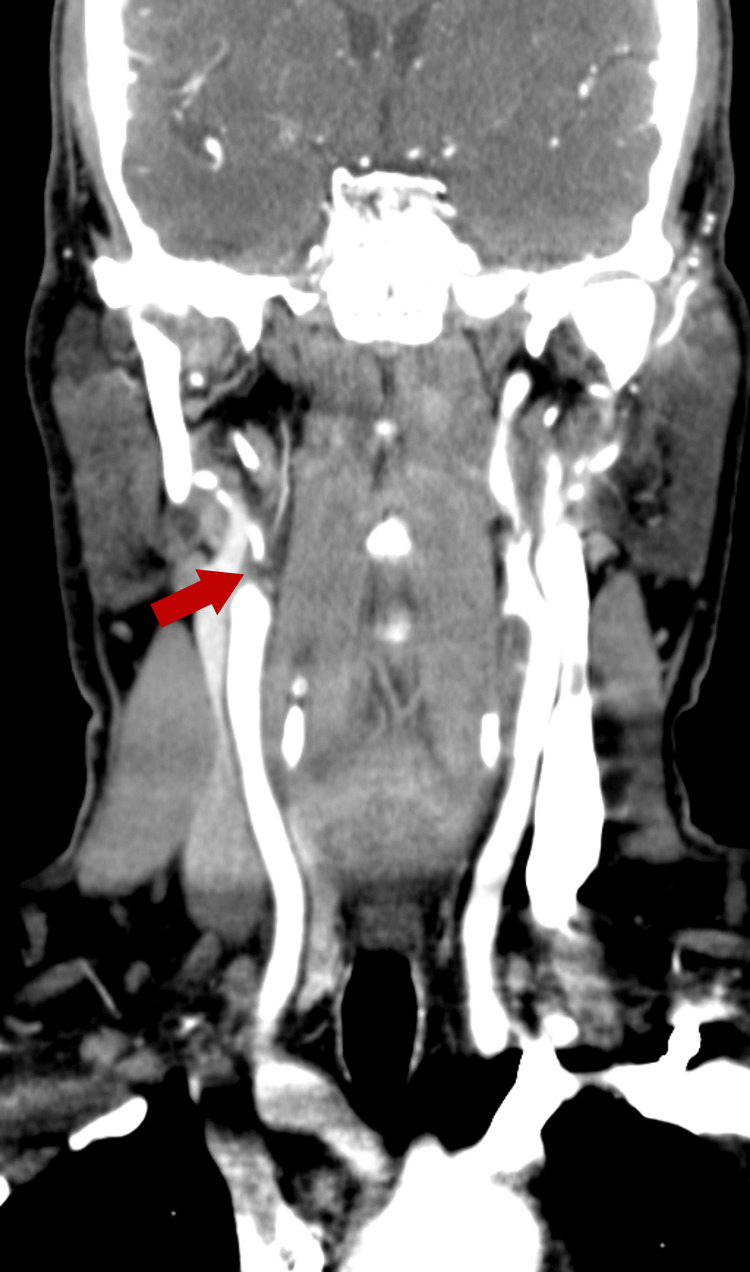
CT angiography. CT angiography demonstrating near-complete occlusion of the right internal carotid artery distal to the carotid bulb, with residual filiform flow (red arrow).
